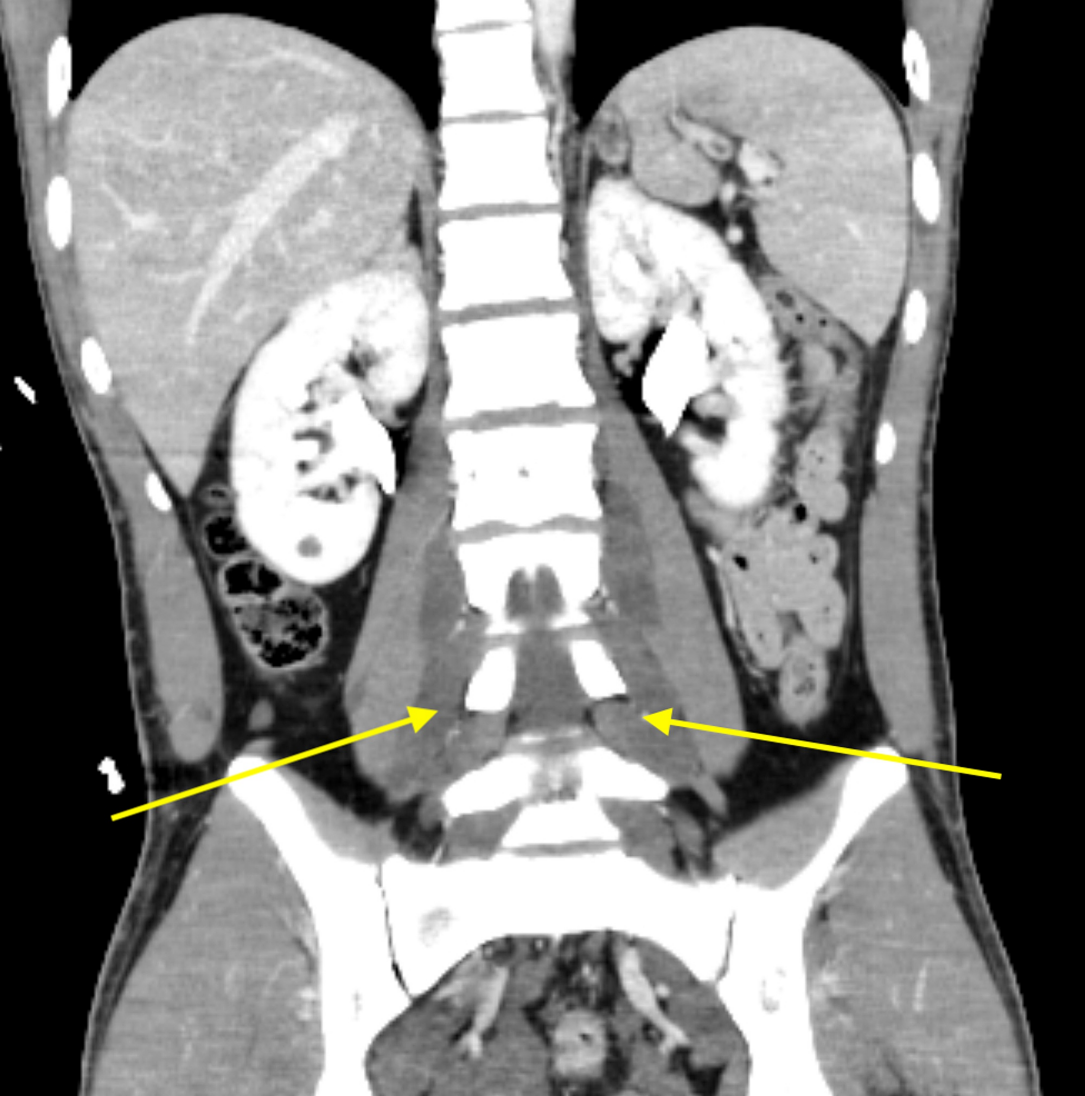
Contrasted coronal CT again demonstrates low-density masses that traverse multiple neuroforamina bilaterally and are characteristic of retroperitoneal plexiform neurofibromas (yellow arrows).
